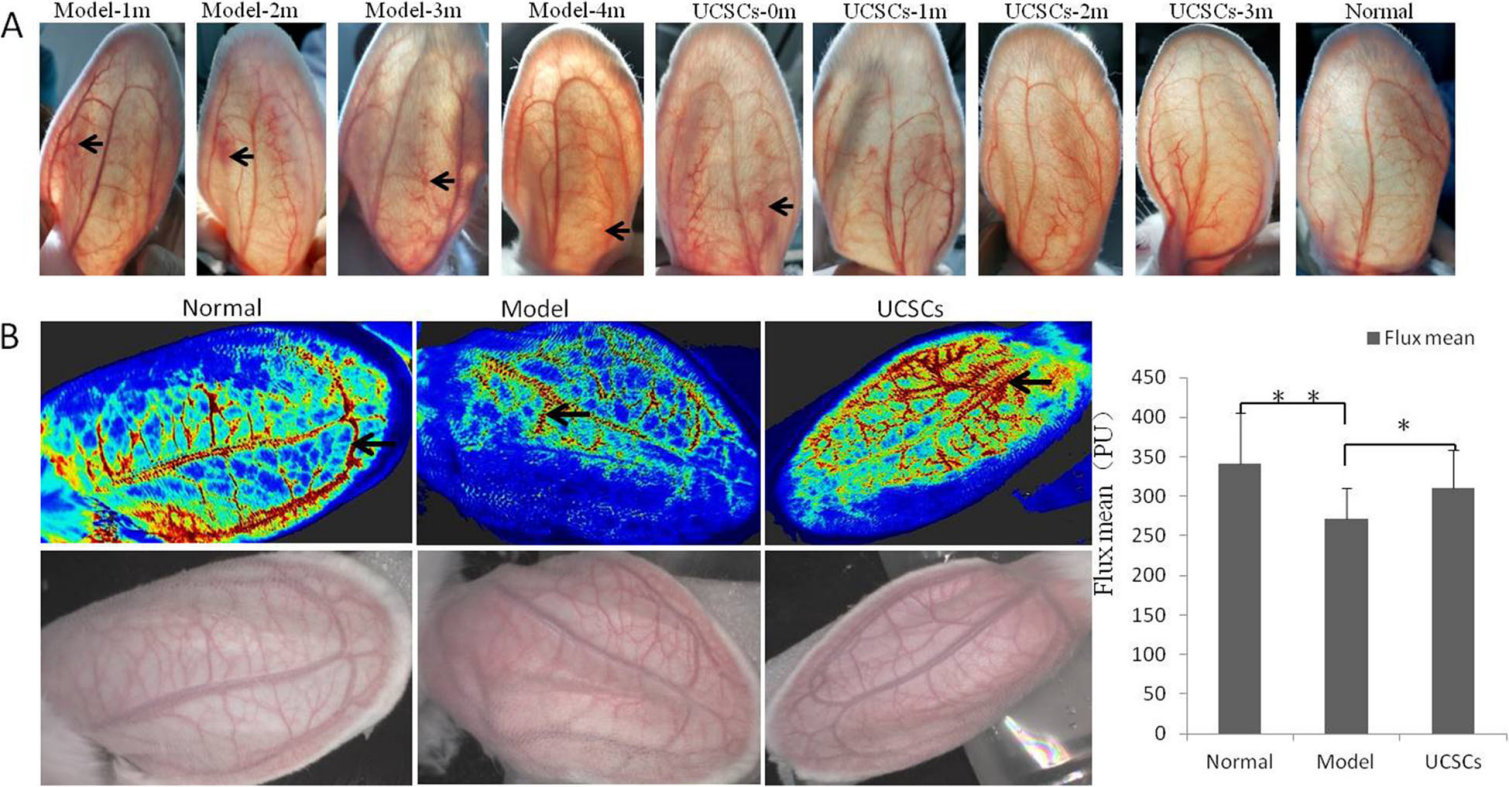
Detection of blood filling of peripheral blood vessels in rabbit ears. A Morphological changes of ear peripheral blood vessels at different time points. The blood flow of the model group was not unobstructed (shown by the arrow) after 1, 2, 3, and 4 months of high-fat diet. B Analysis of laser Doppler flow imaging. Intravascular hemoperfusion (black arrow) in the model group was less than that of the control and UCSCs groups, and the average blood flow filling value (flux mean) was significantly reduced compared with the control and treatment groups. (n = 10 in each group) P* < 0.05, *P* < 0.01, compared with model group
